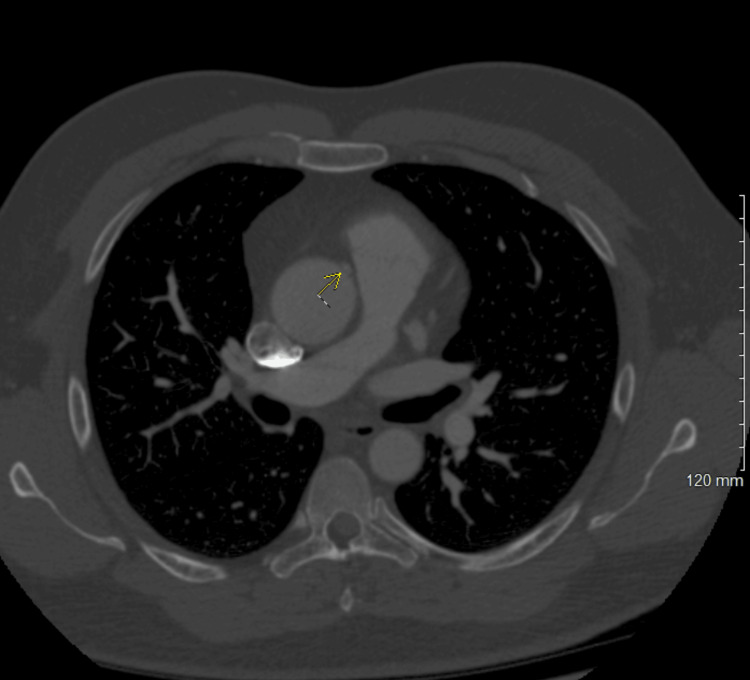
CTA displaying the AORCA (yellow arrow). CTA, computed tomography angiography; AORCA, anomalous origin of the right coronary artery
